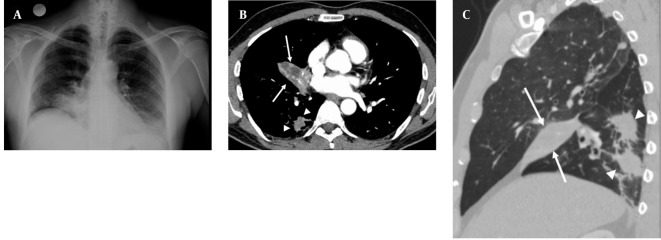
A 50-year-old man presenting with shortness of breath A, Posterior-anterior chest radiograph shows opacity in the right lower lung zone above the diaphragm deleting the right cardiac border; B, Axial CT image shows a mass (arrows) with hypodense areas in the middle lobe and irregular nodules in the right lower lobe (arrow heads); C, Sagittal reformatted CT image shows right middle lobe collapse caused by the mass.
